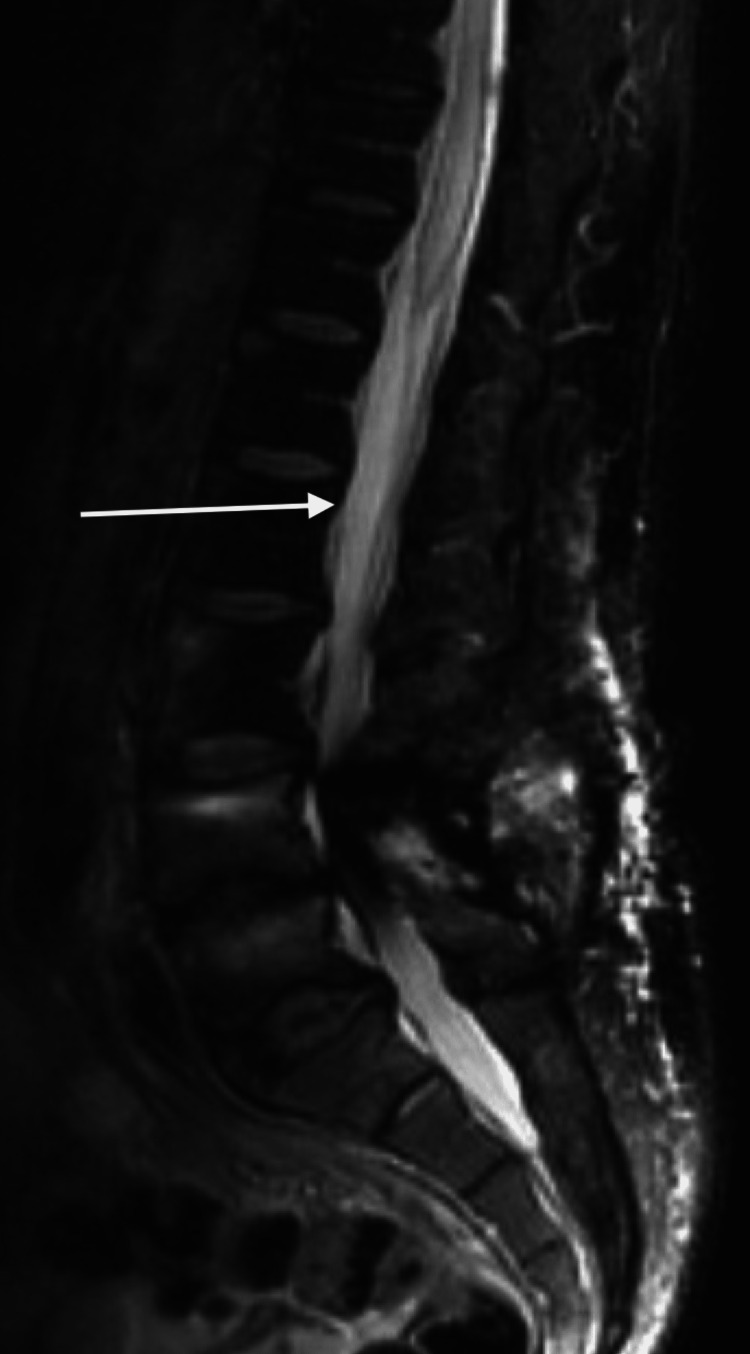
Magnetic resonance imaging of the lumbar spine also showing interval change of extension of the lesion caudally to T5
